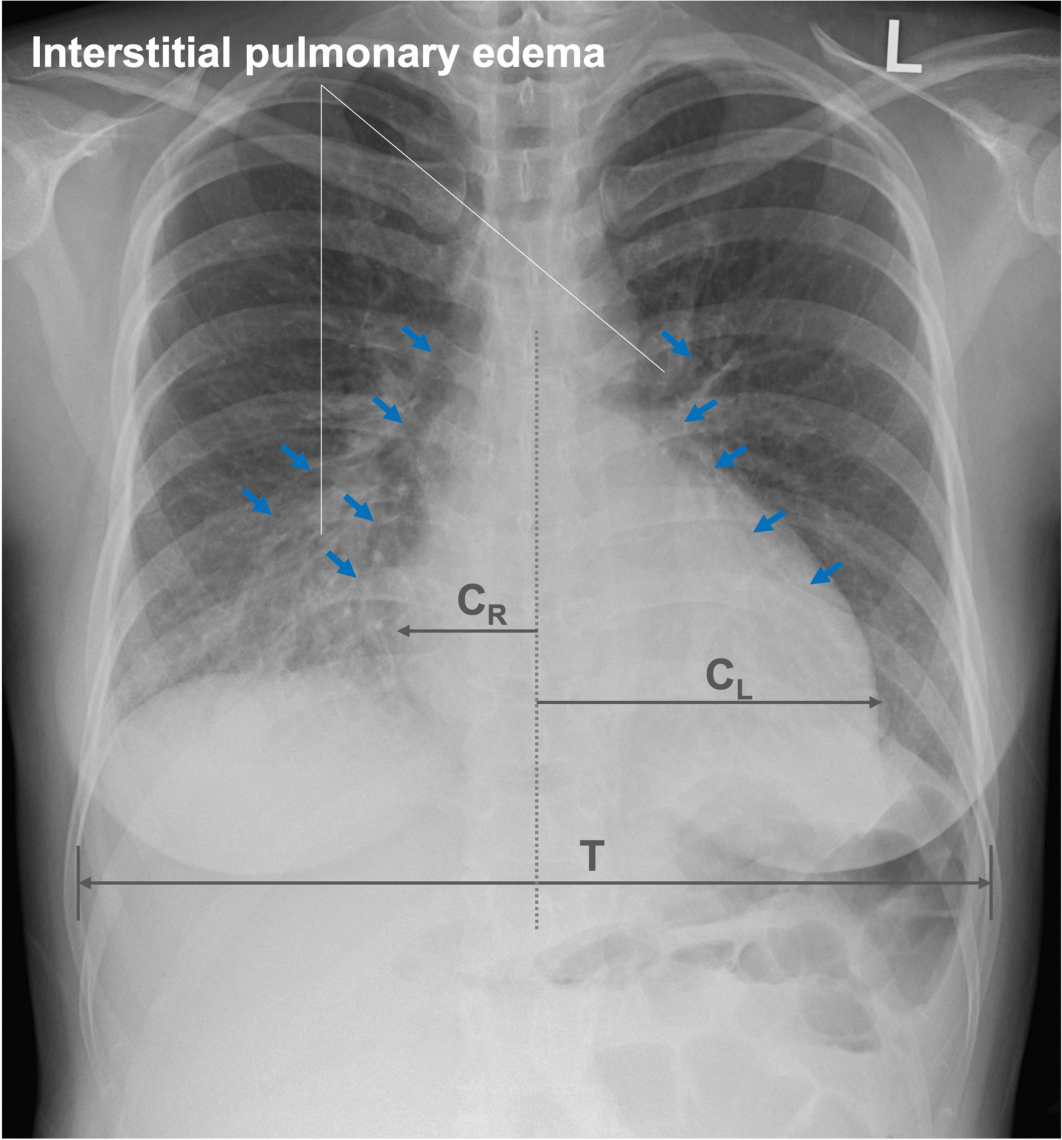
Chest radiograph at presentation The posteroanterior chest radiograph at presentation demonstrates cardiomegaly, with a CTR of 53% (calculated using the formula (C_R_ + C_L_)/T × 100), and bilateral interstitial pulmonary edema, characterized by air bronchograms (indicated by filled arrows). These findings suggest pulmonary congestion due to heart failure (T-CM). CTR, cardiothoracic ratio; C_L_, horizontal left cardiac diameter; C_R_, horizontal right cardiac diameter; T, maximal horizontal thoracic diameter; T-CM, tachycardia-induced cardiomyopathy
